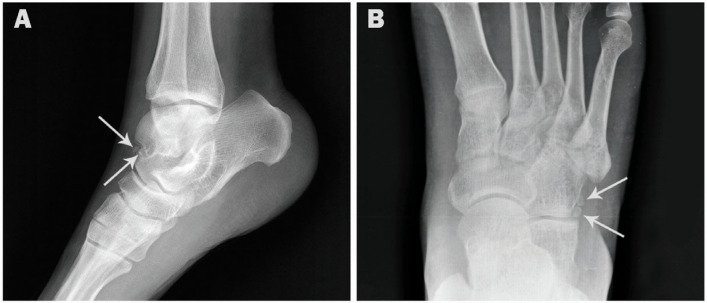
Radiographs showing bipartite ossicles (arrows). (A). Lateral radiograph showing bipartite supratalare in a 44-year-old female. (B). AP radiograph showing bipartite os peroneum in 50-year-old male.
